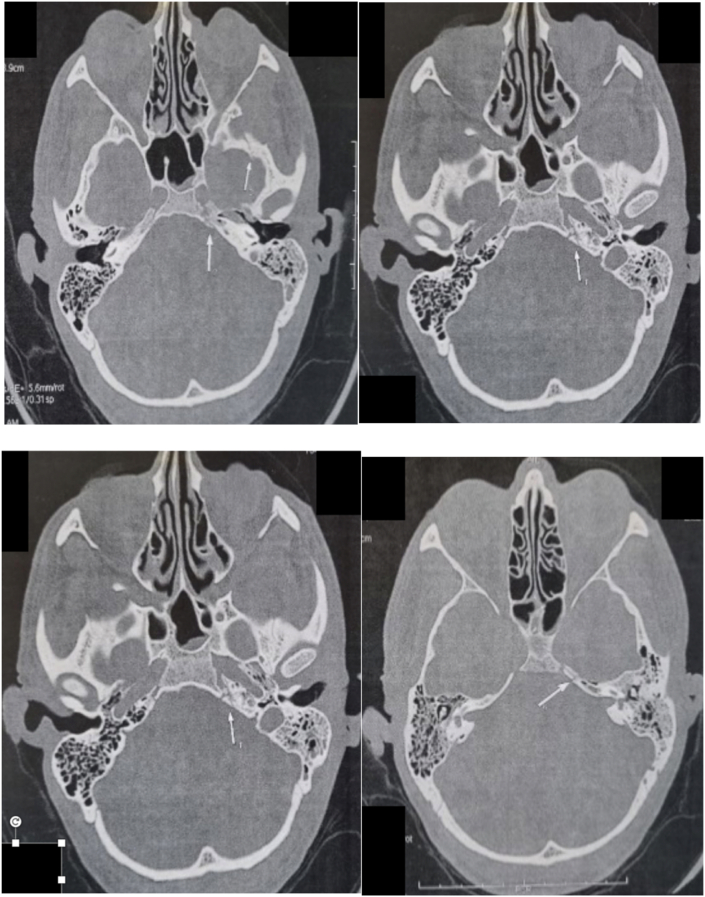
(a and b) Transverse section of cranial CT showed translabyrinthique fracture of the left temporal bone with extension to the left apex petrous.
